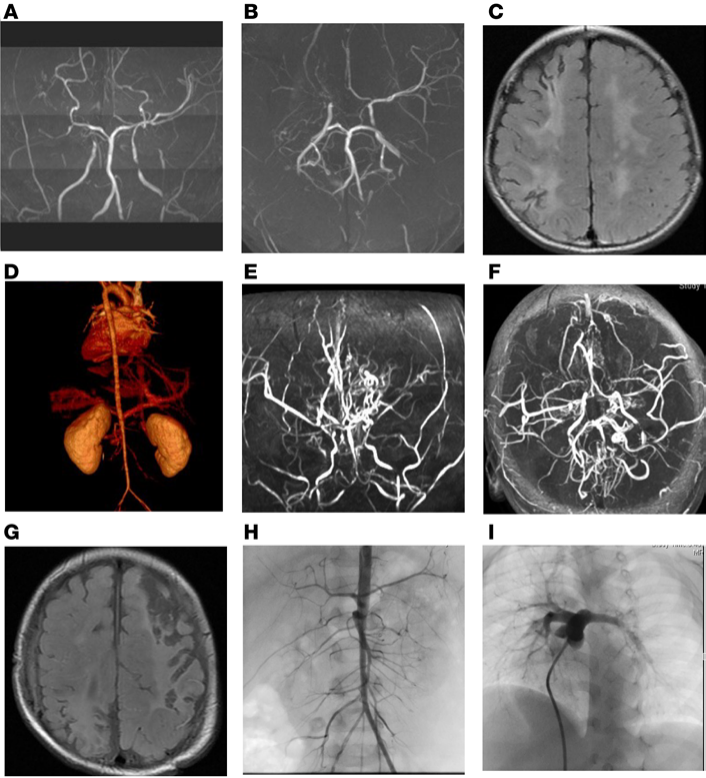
Clinical imaging data of the patients. Upper. Patient 1 at 4 years and 3 months. Magnetic resonance angiography (MRA) frontal (A) and axial (B) views of the cerebral artery show bilateral severe stenosis of the internal carotid artery. The right anterior and right middle cerebral arteries are not detected. Brain atrophy of the right cerebral hemisphere and bilateral high intensity on white matter lesion on FLAIR-MRI (C). Three-dimensional CT angiography at 2 years and 10 months shows narrowing across the thoracoabdominal aorta to the iliac artery (D). Middle. Patient 2 at 1 year and 5 months. MRA frontal (E) and axial (F) views of the cerebral artery show bilateral severe stenosis of the internal carotid artery and bilateral vertebral artery to basilar artery stenosis. Brain FLAIR-MRI at 1 year and 8 months shows old cerebral infarcts in the left frontoparietal and right temporal and occipital lobes (G). Angiography at 1 year and 11 months showed stenosis of the abdominal aorta and bilateral renal arteries (H) as well as peripheral pulmonary artery stenosis (I).
